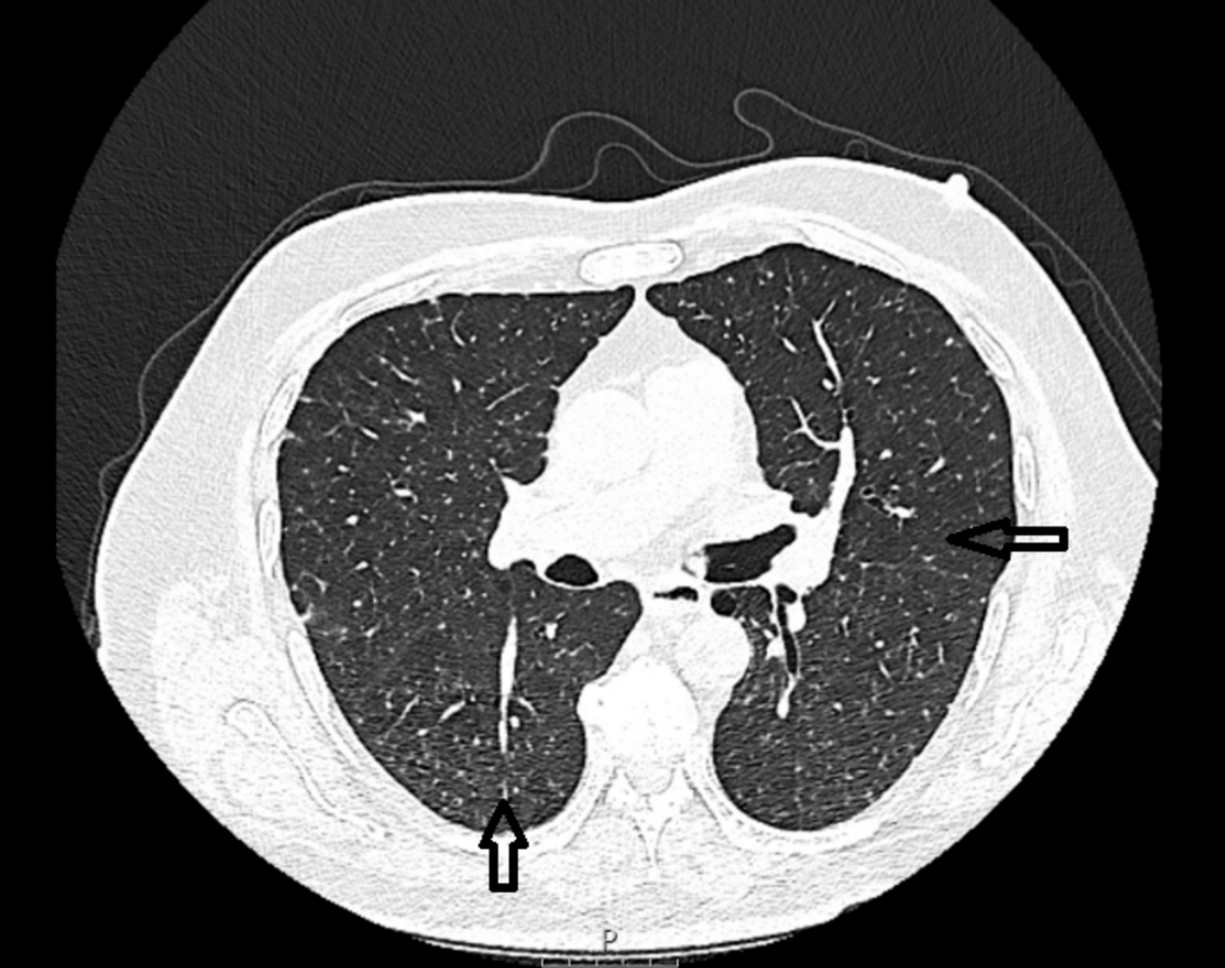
Chest CT: Resolution of the ground-glass opacities and early interval improvement in the miliary micronodules (arrows) CT: computed tomography
